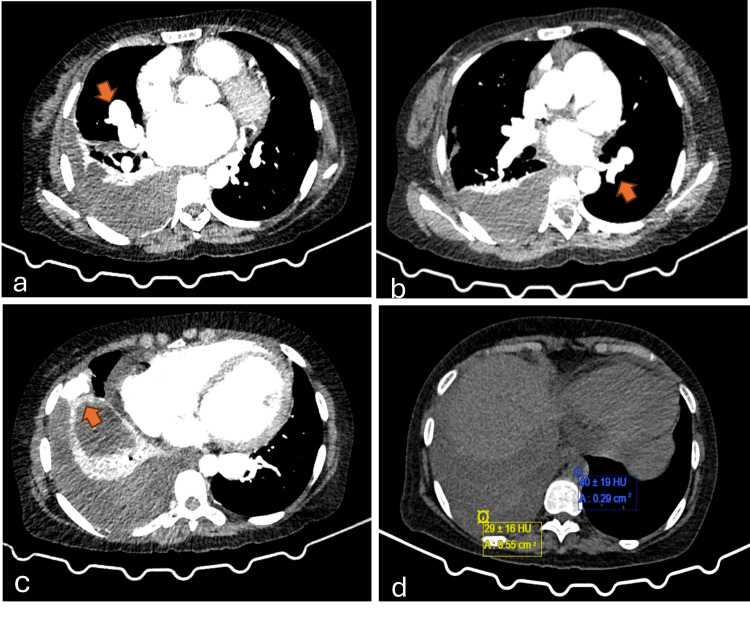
CTPA showing dilated and tortuous pulmonary vessel (arrow) (a), anomalous communication between left pulmonary vein and branch of left pulmonary artery (b), dilated subpleurally located dilated pulmonary vessel (arrow) (c), Non-contrast CT of thorax- Ratio of attenuation values in pleural fluid and aortic blood of 0.72 (threshold: ≥0.56). CTPA: computed tomography pulmonary angiography, HU: Hounsfield units.
